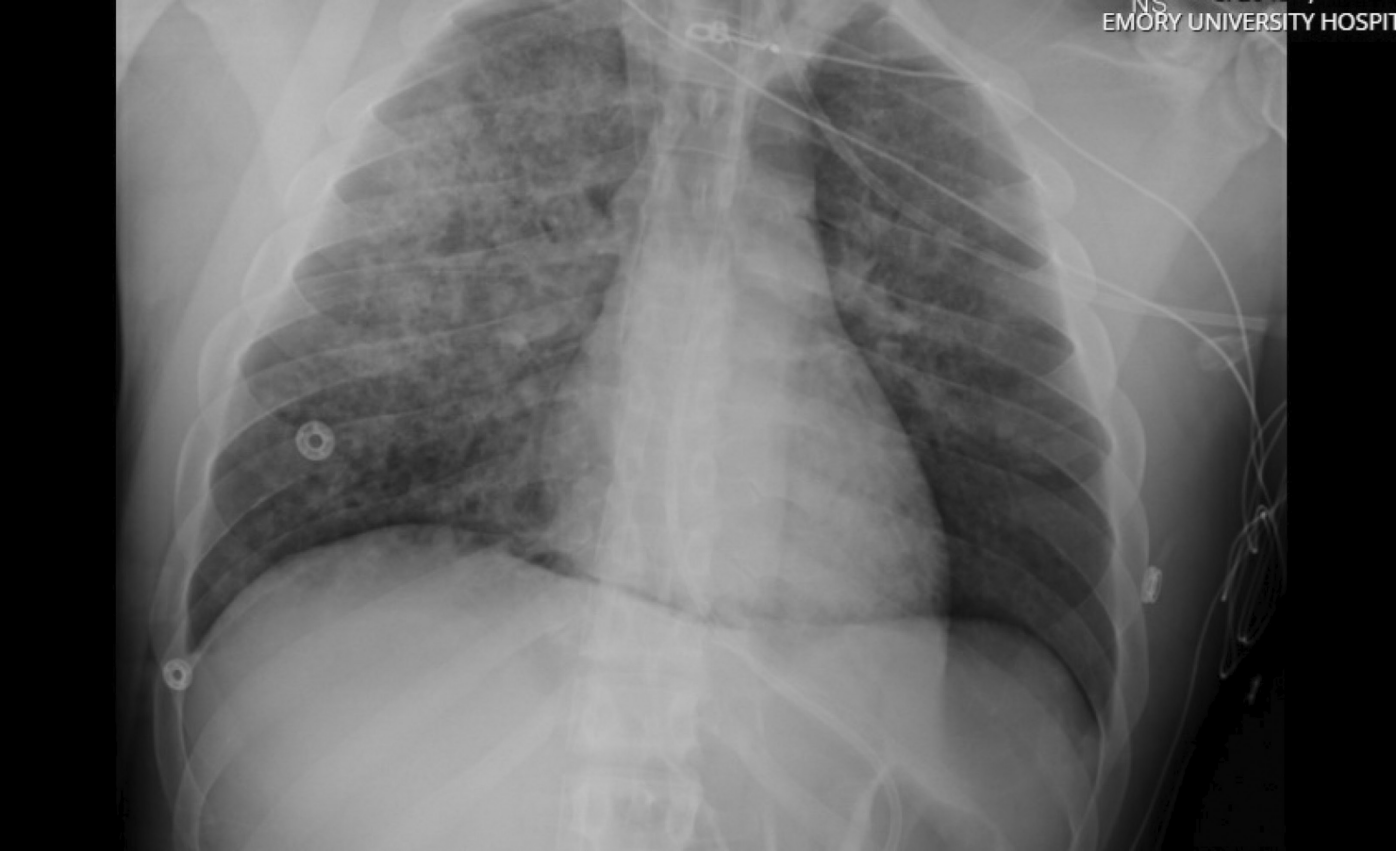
Portable chest radiograph hospital Day 3 (less than 48 h after cannulation). This radiograph demonstrates persistent extensive bilateral interstitial/airspace opacities with subsequent removal of endotracheal tube.
